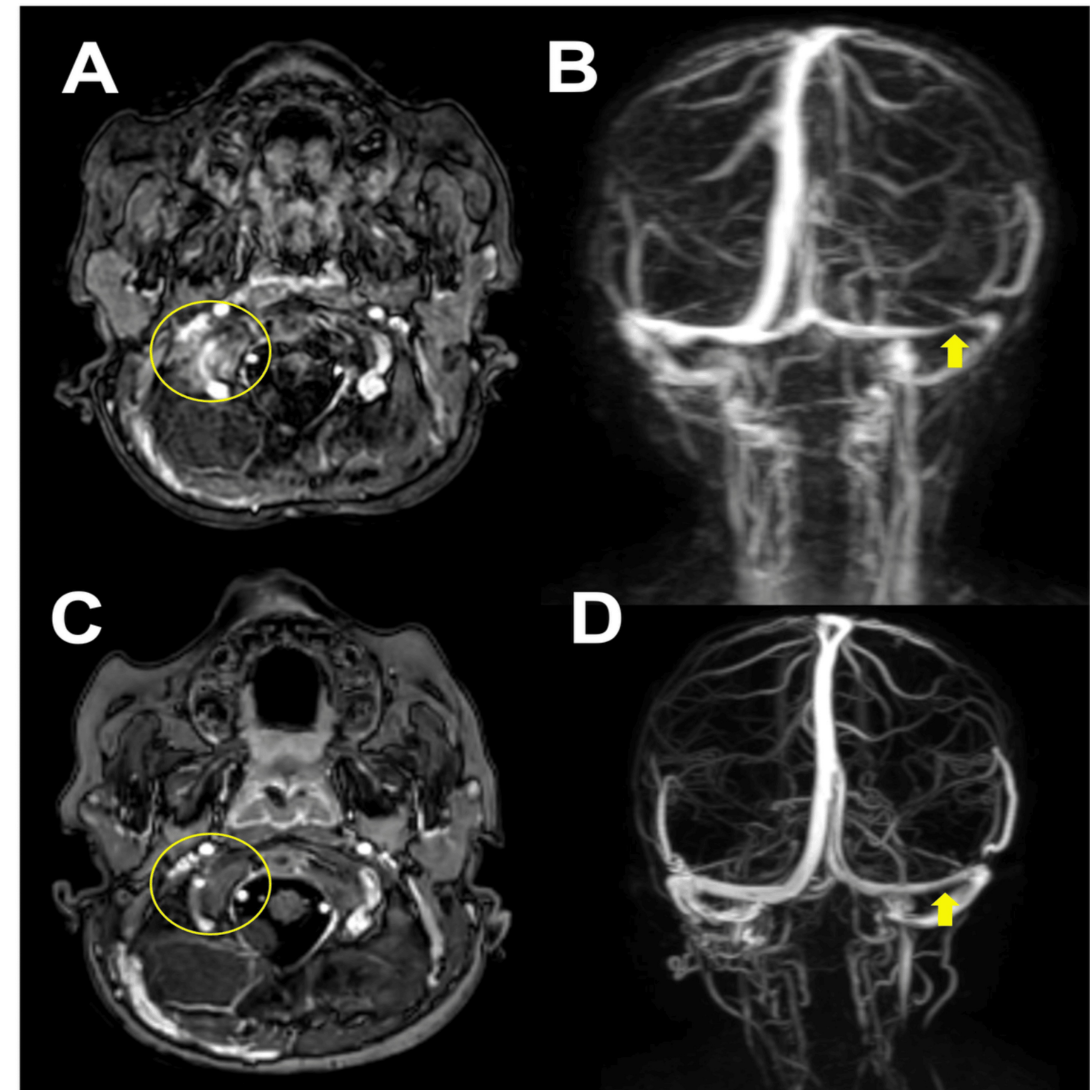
Magnetic resonance imaging and venogram. (A) The circle shows cerebellitis. (B) The arrow indicates partial venous thrombosis of the transverse sinus. (C, D) The circle and arrow show resolution of venous thrombosis.
